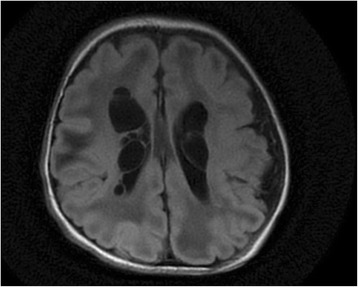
Brain MRI of patient 2. FLAIR images of the brain of patient 2 showing multiple periventricular cystic lesions in addition to attenuated signal in the subcortical white matter
